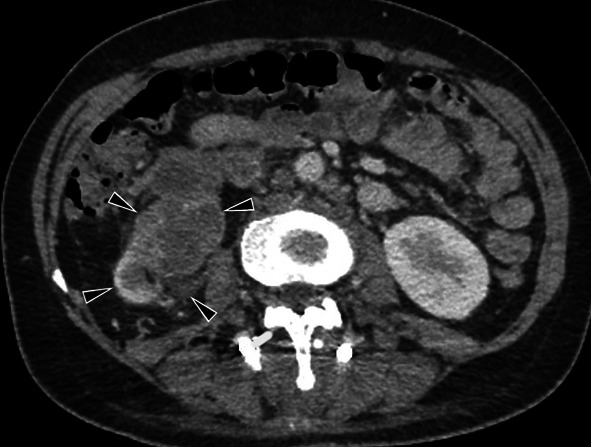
Contrast-enhancement CT scan of a 48-year-old female with renal tuberculous show debris collection within dilated calyces and parenchymal destruction (with cortical thinning)
